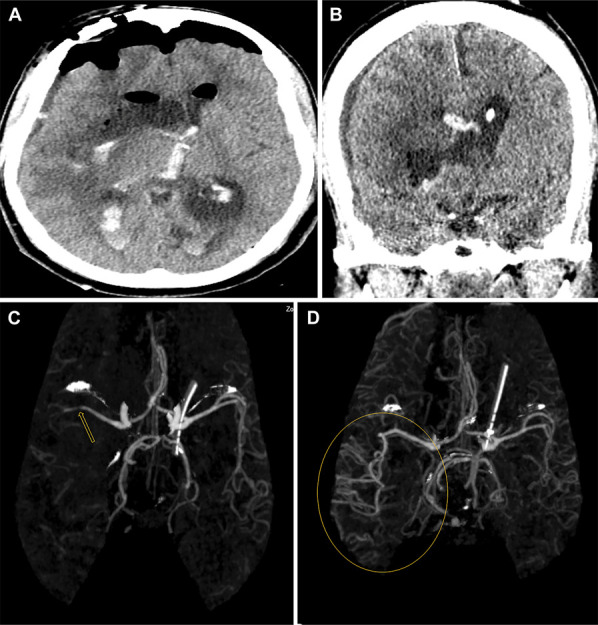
Postoperative CT and CTA scans show improvement in midline shift and recanalization of MCA after hematoma evacuation. A, In the early postoperative period, the axial CT scan reveals an improvement in midline shift. Despite a slight hemorrhage visible in the coronal section within the ventricle, the external ventricular drain placement is appropriate and provides effective ventricular drainage (arrow) B, After the postoperative endoscopic hematoma evacuation, a return to normal filling is observed in the MCA vessel C, and there is an improvement in blood flow in the distal regions (circle). D, In the preoperative CTA scan a suspicious appearance of a large vessel occlusion is observed just before the bifurcation (indicated by an arrow) of the right MCA's distal segment. There is a noticeable reduction in blood flow in the distal MCA territory. CT, computed tomography; CTA, CT angiography; MCA, middle cerebral artery.
